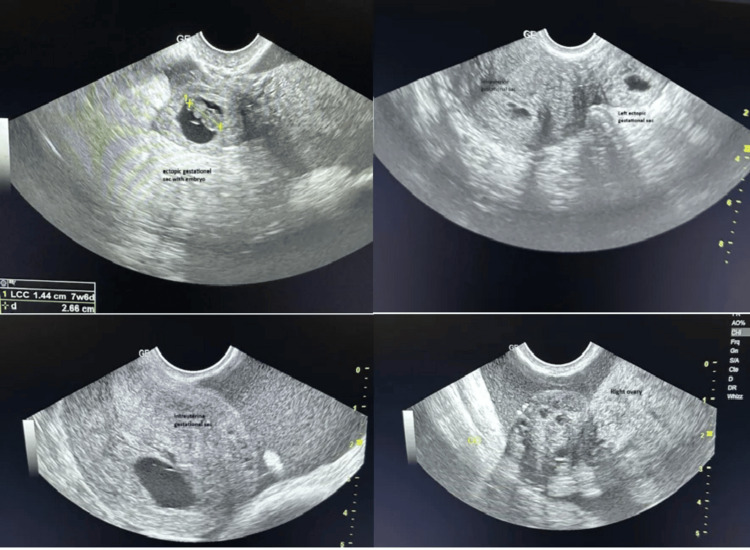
Transvaginal ultrasonography showing heterotopic pregnancy at seven weeks + six days
